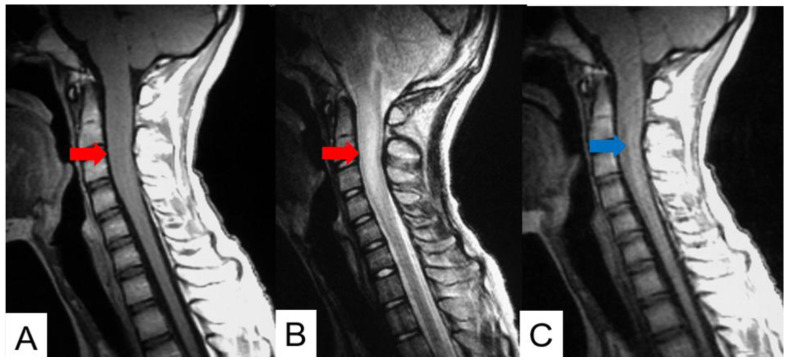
Fourteen M, spinal astrocytoma, grade 3. (A) T1-weighted midsagittal image, (B) T2-weighted midsagittal image, and (C) enhanced T1-weighted midsagittal image. Red arrows indicate tumor and blue arrow shows tumor enhancement.
